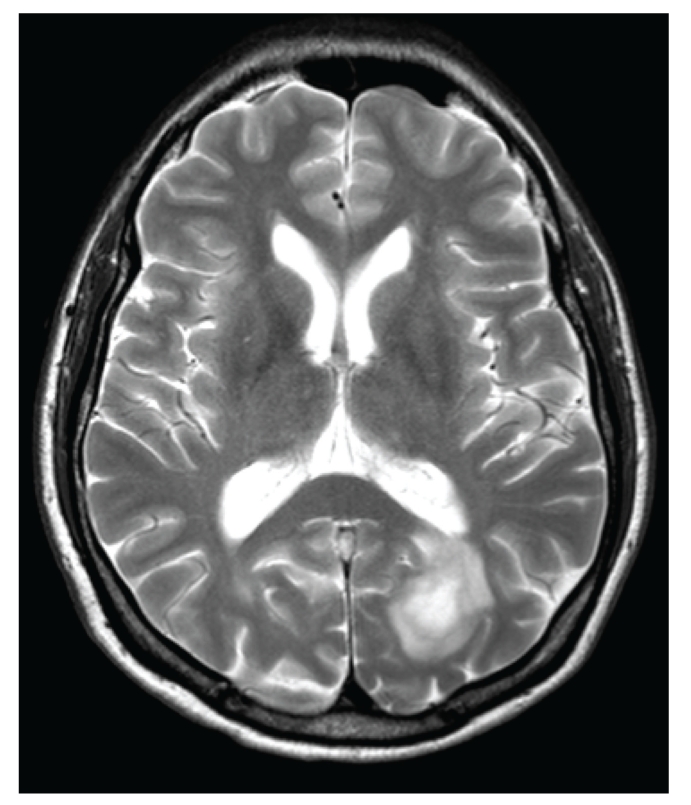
Axial T2-weighted brain MRI shows a large left-sided hyperintense occipital lesion.
